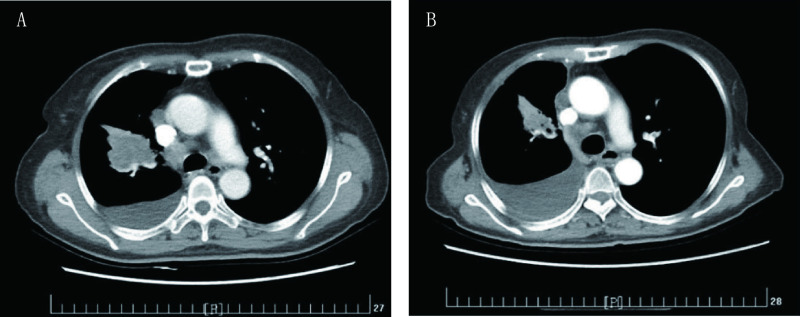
第一次培美曲塞应用。A：第一次应用前基线检查（2015年11月5日）；B：第一次应用最佳疗效（2016年3月4日） The first pemetrexed challenge. A: The baseline CT image before the first pemetrexed challenge (Nov. 5, 2015); B: The best response CT image after the first pemetrexed challenge (Mar. 4, 2016)
